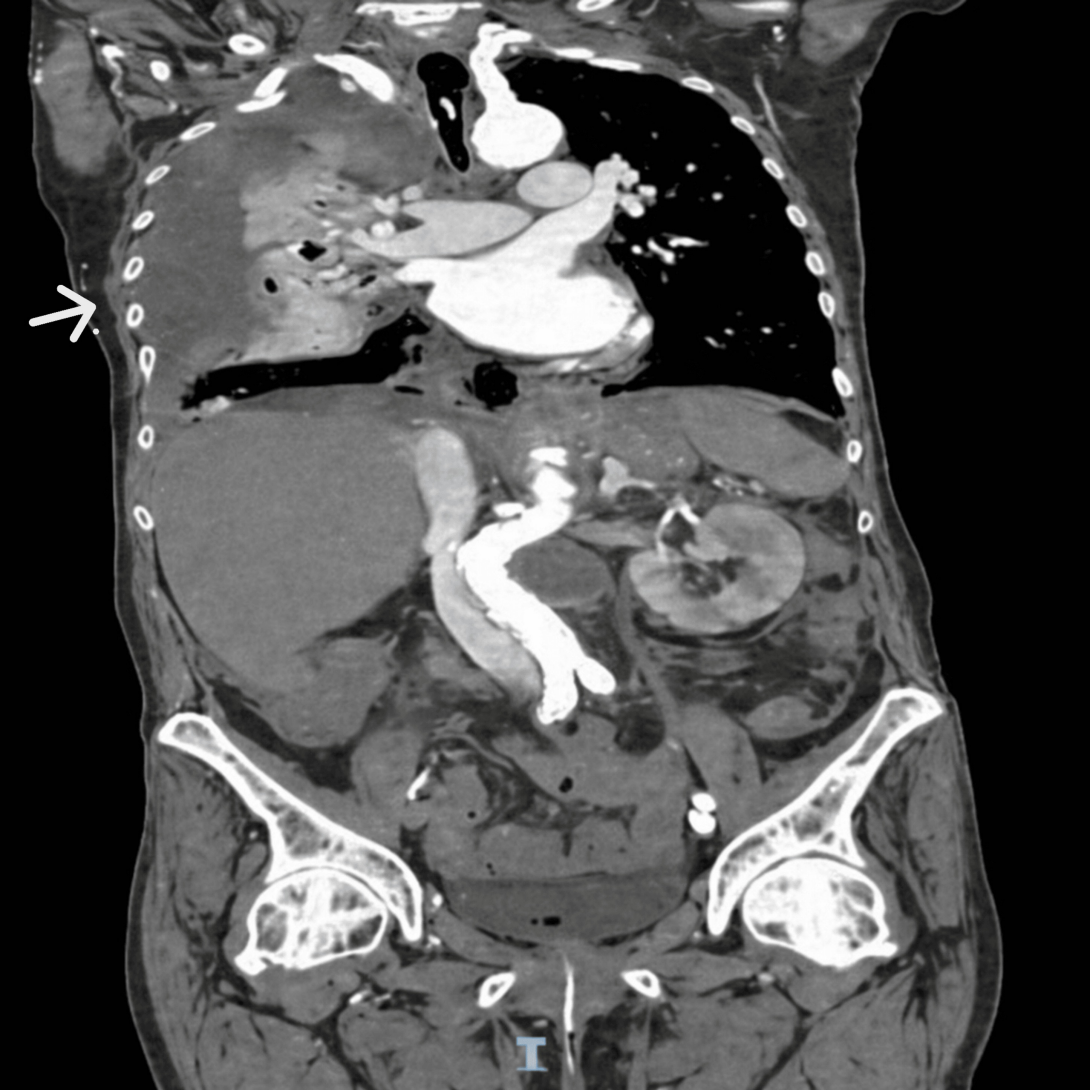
Coronal CT (venous phase) reconstruction demonstrates the extent of right-sided hydropneumothorax and minimal left pleural effusion. The posterior mediastinal air is again visualized (arrow), supporting the suspicion of esophageal rupture.
